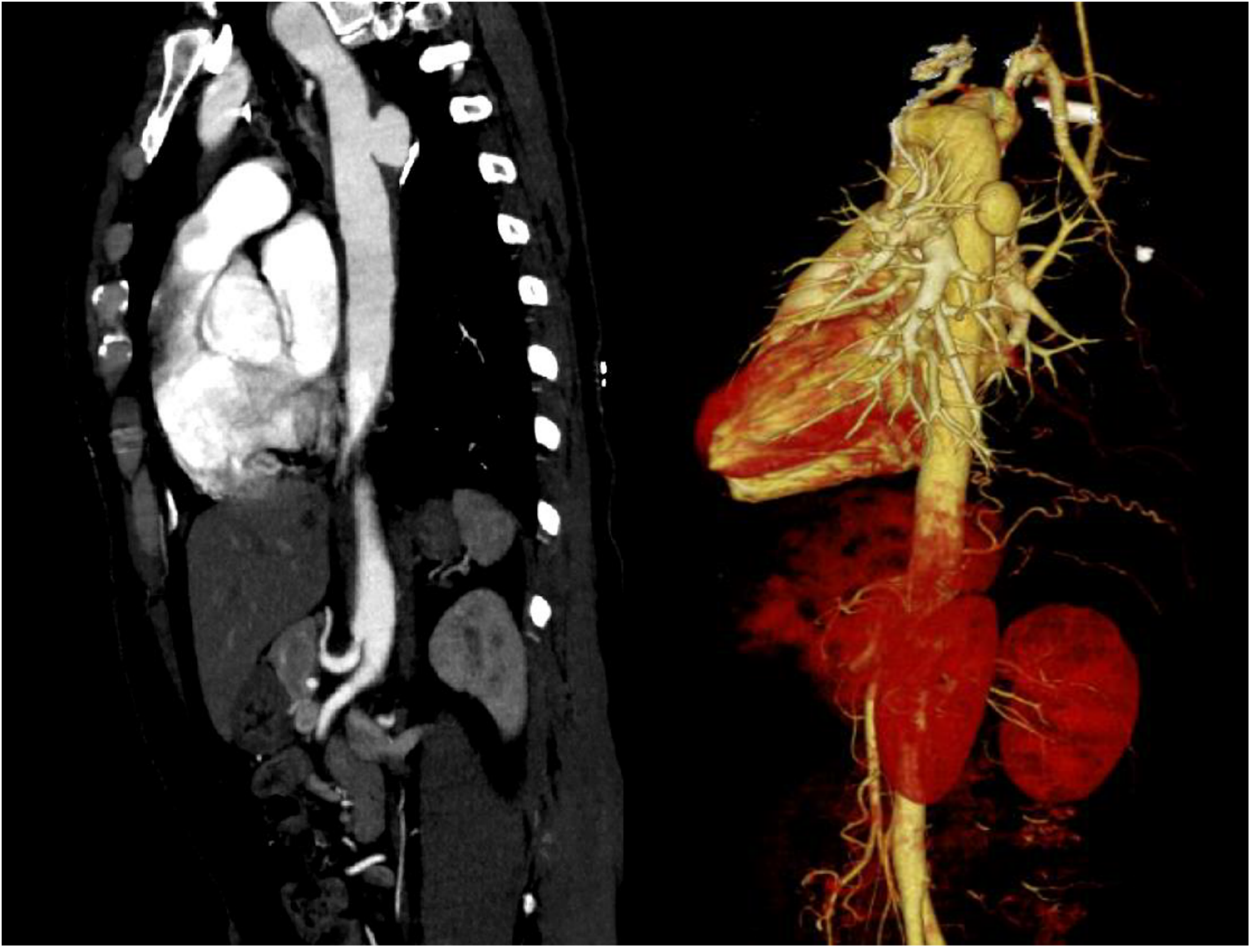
CTA indicated that aortic double arch malformation with suspected development of dissection in the descending aorta.
